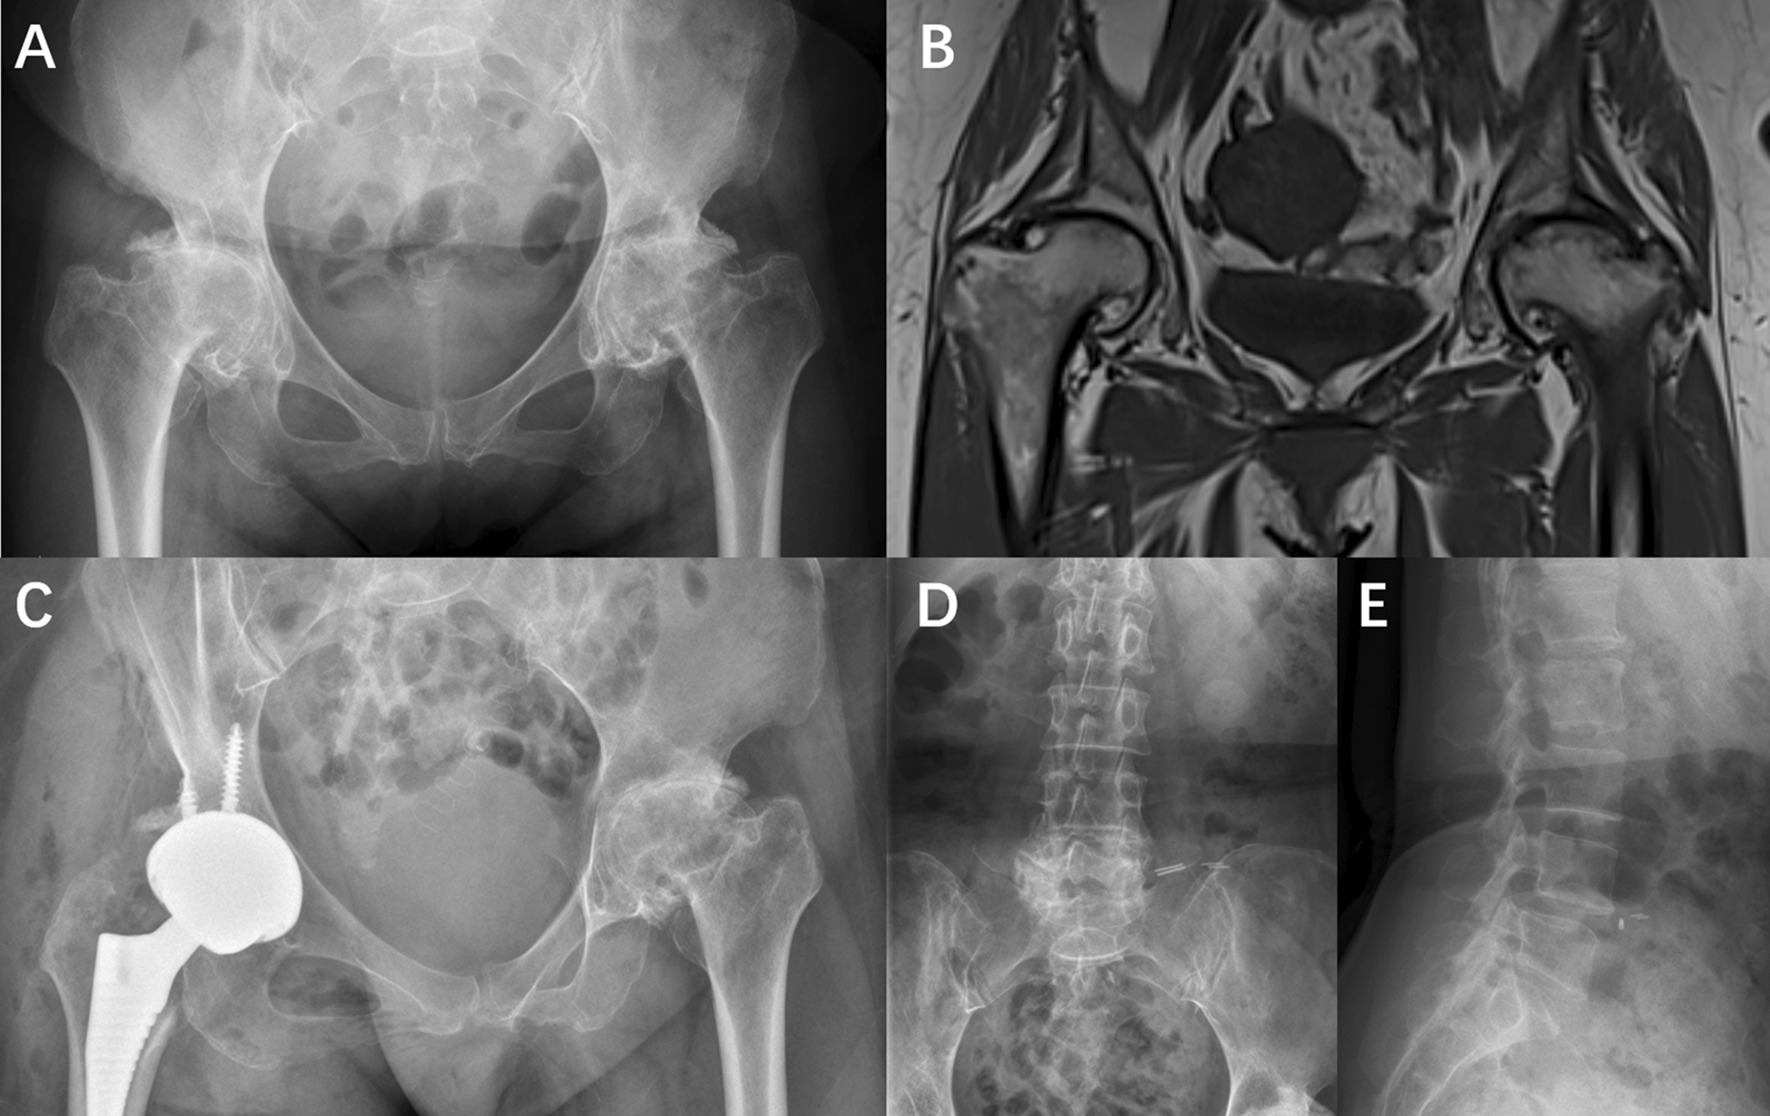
A, B The X-ray and MRI revealed that collapsed femoral heads with cystic degeneration, and premature hip osteoarthritis in both hips of the proband (IV9). C Post-operation radiograph. D, E The X-ray of the patient's spine indicated normal vertebrae
